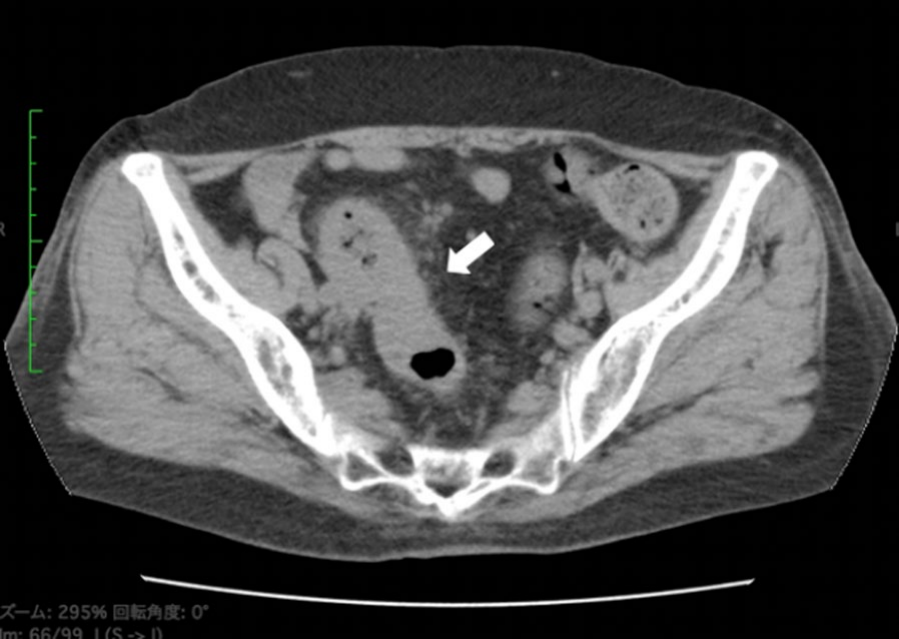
CT finding at first visit. CT scan showed increased adipose tissue concentration around sigmoid colon and thickening of sigmoid colon wall (arrow). It caused bowel obstruction and was suspected sigmoid colon cancer
